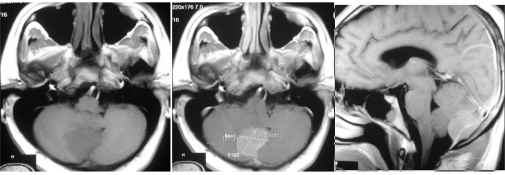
MRI study demonstrated a homogenously enhanced intracranial mass of the posterior fossa. (left) hypointense mass on T1-weighted image, (centre) well-absorbed contrast agent on T1 with contrast agent, (right) note the hyperintense line in the middle of the mass of the sagittal view.
